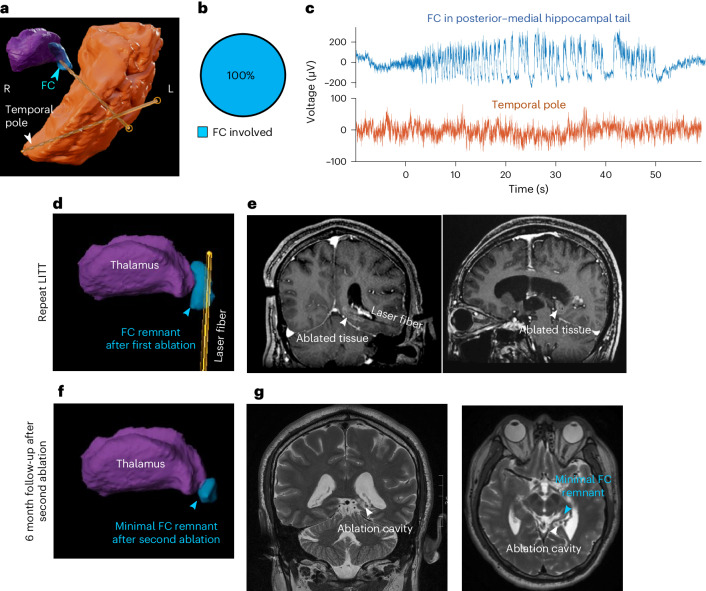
The FC is a viable treatment target in humans. a, Reconstruction of sEEG electrodes (yellow) in remnants of the hippocampal tail (blue), as well as in the temporal pole (orange) of patient 6 who had a previous amygdalohippocampectomy. The thalamus is shown in purple. b, All seizures recorded from this patient had FC involvement (*n* = 117 out of 117 seizures). c, LFP trace for a representative seizure originating from the posterior–medial hippocampal tail remnant (onset time at 0 s), as recorded by electrodes in the FC (blue) and in the temporal pole (orange). d,e, Three-dimensional reconstruction (d) and intraoperative in-line and orthogonal slice of post-contrast T1 MRI (e) showing a laser fiber inserted in the lateral edge of the hippocampal tail to avoid heat spread into the thalamus. The contrast-enhancing lesion appears to include the entirety of the remnant. f,g, Three-dimensional reconstruction (f) based on coronal and axial T2-weighted MRI (g) at 6 months following the second ablation, with a small residual FC adjacent to the lateral geniculate nucleus of the thalamus.
